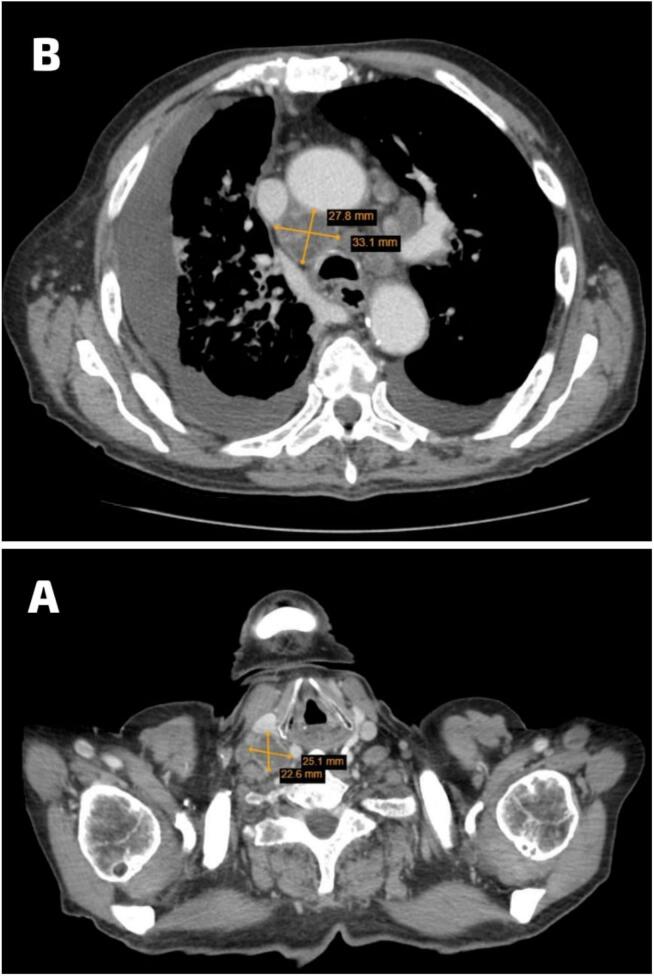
Axial CT scan with IV contrast showed A. multiple enlarged pathological lymph nodes in the right supraclavicular level B. Multiple enlarged mediastinal and hilar lymph nodes with central necrosis.
